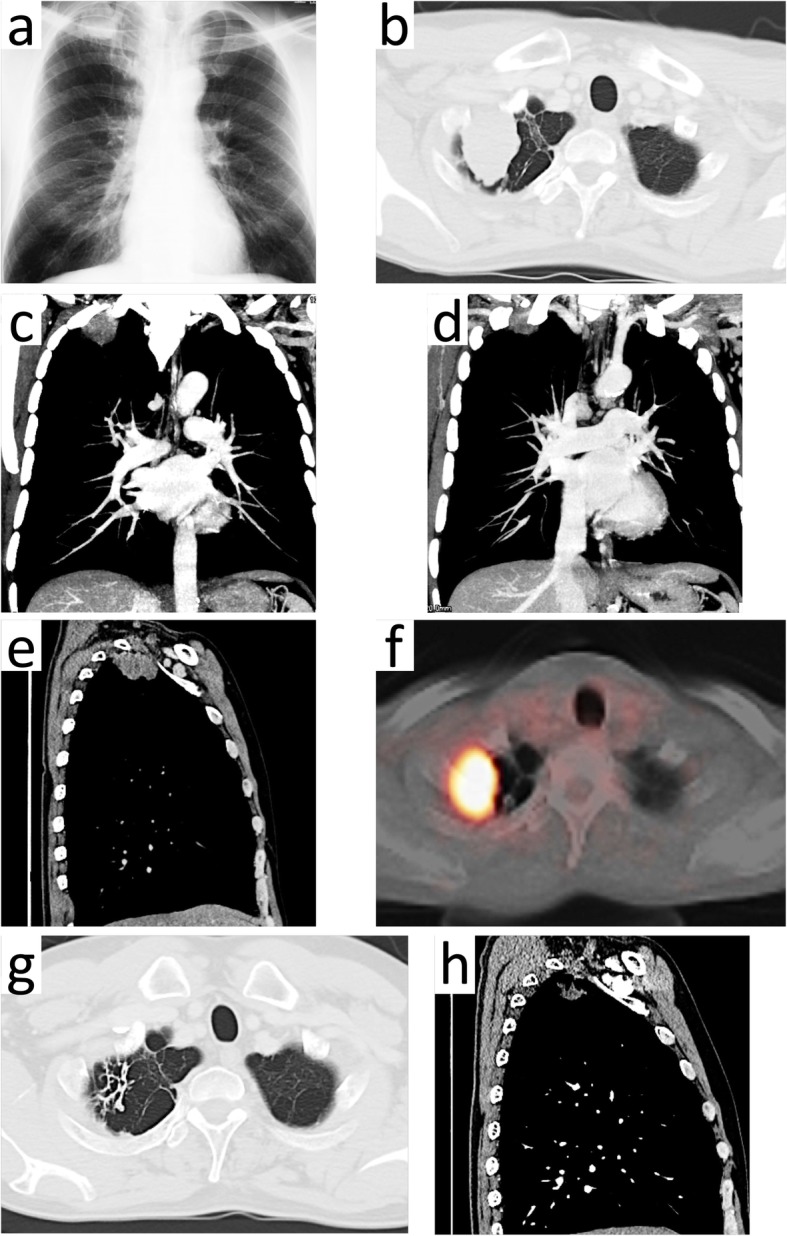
Radiologic imaging of the chest. a Chest X-ray showed a tumoral shadow at the right apex of the upper-lung field. b Computed tomography (CT) of the chest (pulmonary window): a 56 × 30 × 25-mm mass was identified at the right superior sulcus. c, d Coronal chest CT (mediastinal window): the mass was suspected to have invaded the chest wall at the right first and second intercostal space and the subclavian vein. e Sagittal chest CT before chemoradiotherapy (mediastinal window). f Fluorodeoxyglucose-positron emission tomography (FDG-PET). The mass showed a high FDG uptake (SUVmax 13.5). g Chest CT (pulmonary window) performed after chemoradiotherapy revealed the remarkable reduction of the tumor. h Sagittal chest CT after chemoradiotherapy (mediastinal window). The reduction of the mass to 19.5 mm in long diameter was recognized. In addition, it seemed that the contact between the tumor and the chest wall had been released
